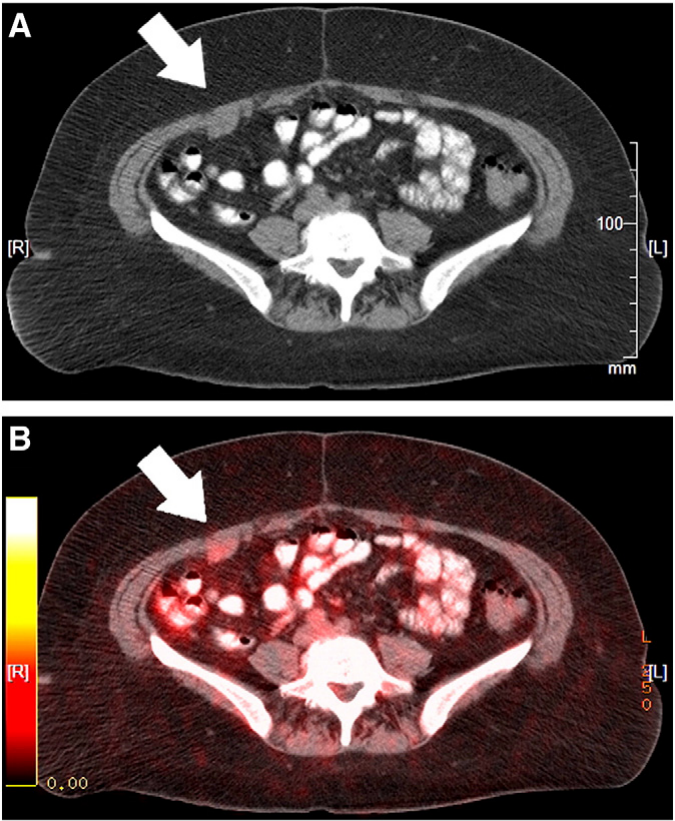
A: CT scan with IV contrast, coronal section at L5 showing anterior peritoneal wall implants on the patient’s right. B: PET scan showing metabolic hyperactivity in these same implants.
